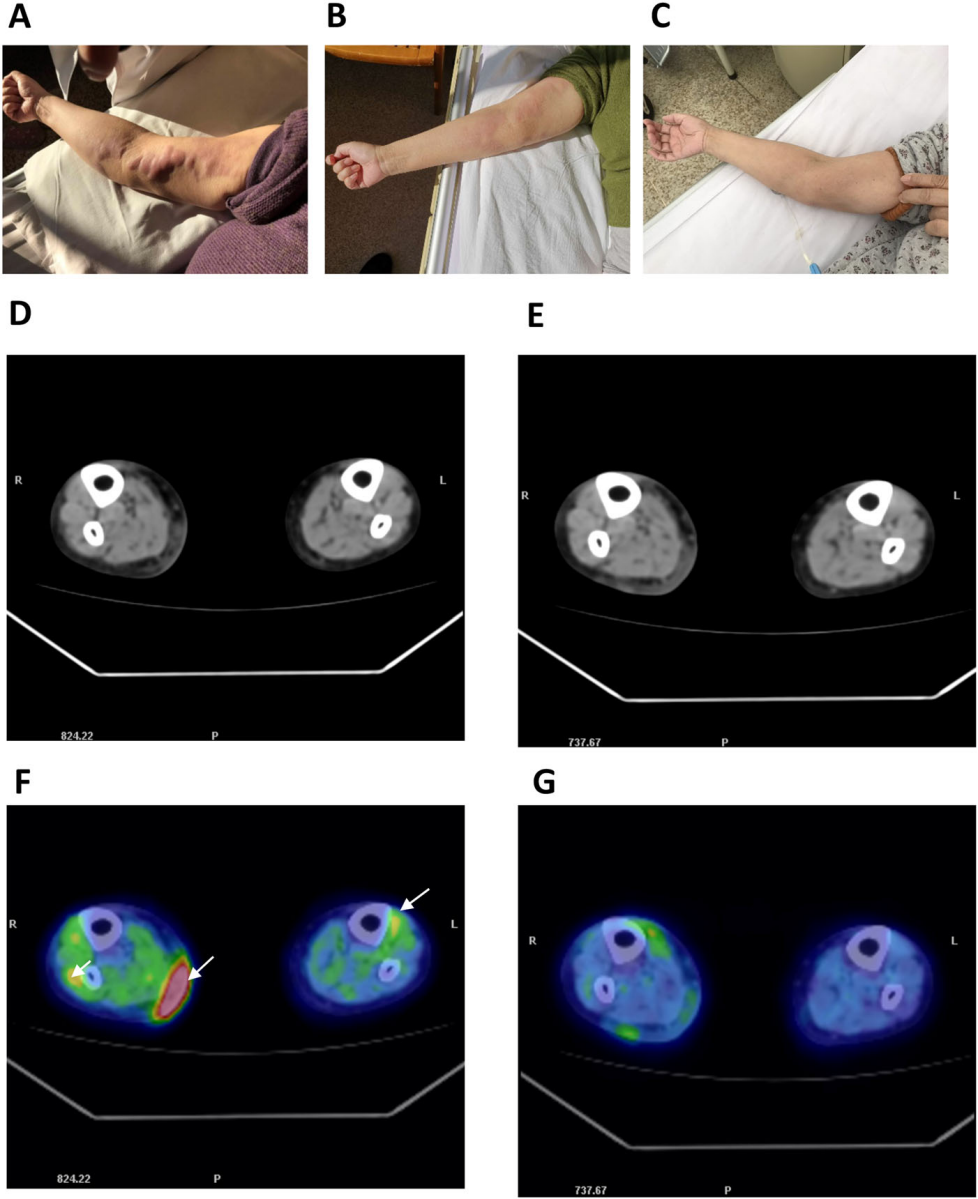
Comparison of DLBCL cutaneous lesions before and after treatment with Chidamide plus Rituximab. a–c Visual results, taken by camera. d, e CT images. f, g PET-CT images. a, d, f Images of patient with multiple cutaneous lesions before the treatment with Chidamide plus Rituximab. The arrows in ‘F’ indicate high-density masses under the skin and in the muscles.
